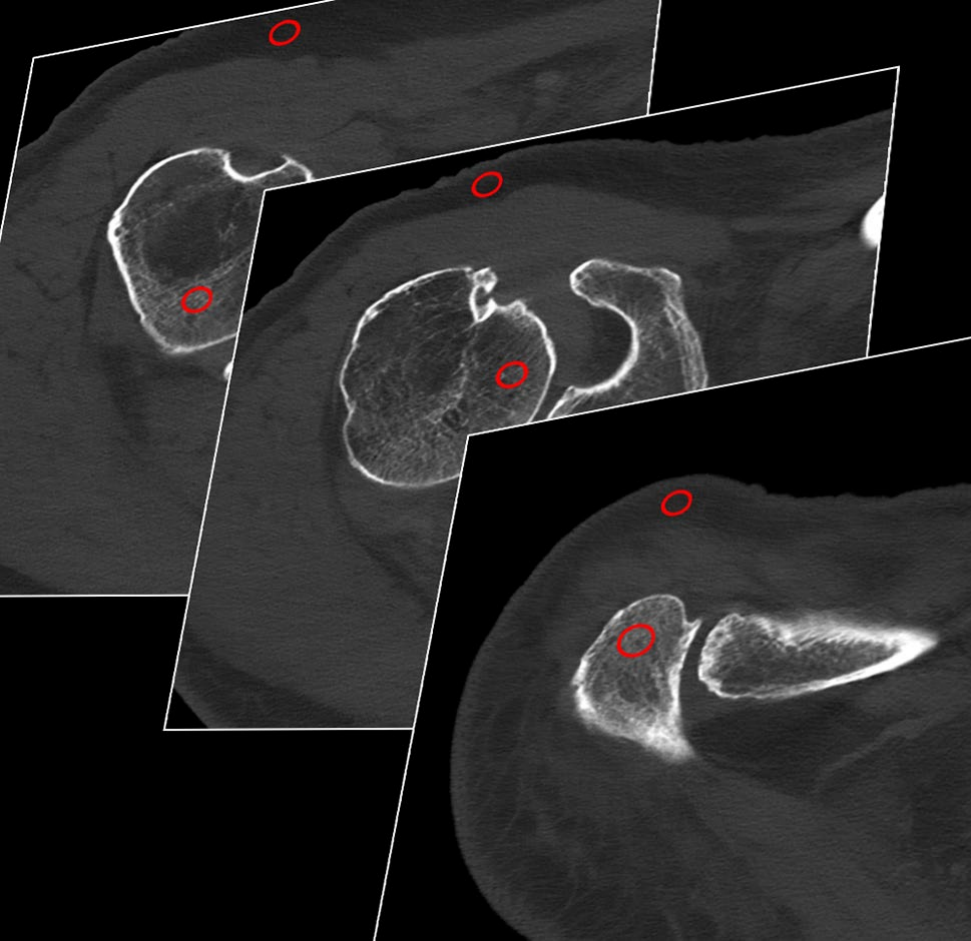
CT number measurements within regions of interest placed in the cancellous bone of the shoulder as well as in adjacent subcutaneous fat tissue on three consecutive axial CT slices.
